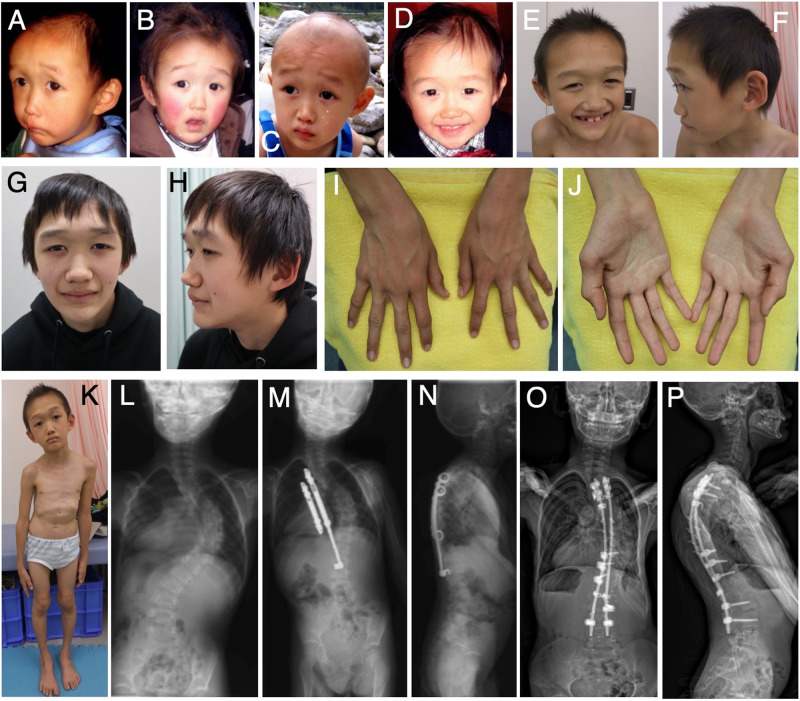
Clinical images of the proband. Facial photographs of the proband at the following ages: 1 year (A), 2 years (B), 3 years (C), 4 years (D), 8 years (E, F), and 16 years (G, H). Images of the proband’s hands at the age of 16 years (I, J). A full body image of the proband at the age of 8 years (K). Radiographs of the proband’s spine at the age of 2 years (L; before surgery), 5 years (M, N; after vertical expandable prosthetic titanium rib implantation), and 12 years (O; after final fixation surgery).
